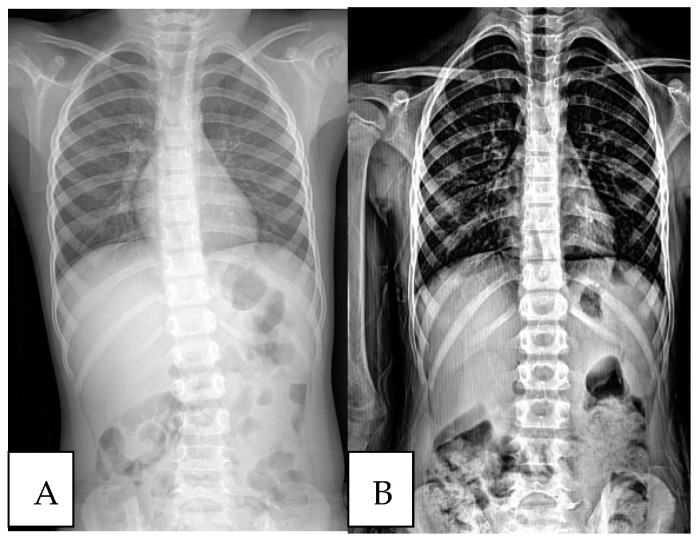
(A) Chest roentgenograph of another patient with a scoliotic attitude detected during preoperative preparation. (B) Post-adenoidectomy scoliosis roentgenograph of the same patient.
